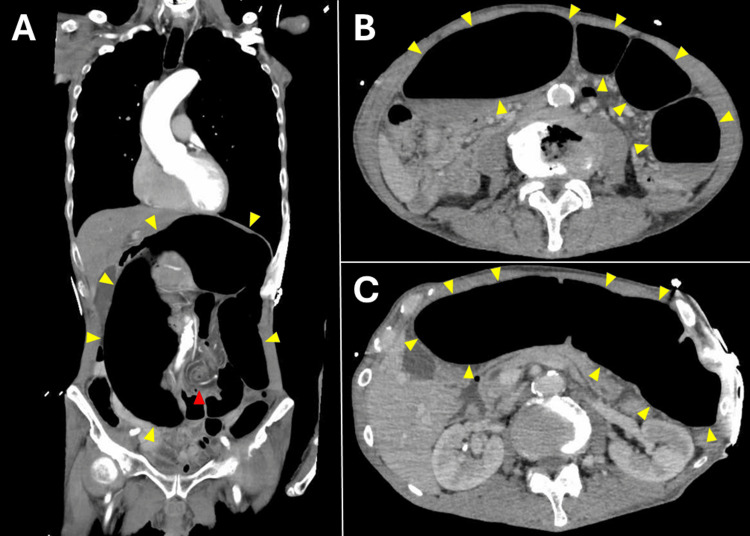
CT scan (A) Coronal view; (B, C) Axial view. A whirl sign (red arrow head) and distended colon (yellow arrow heads) were consistent with sigmoid volvulus.
